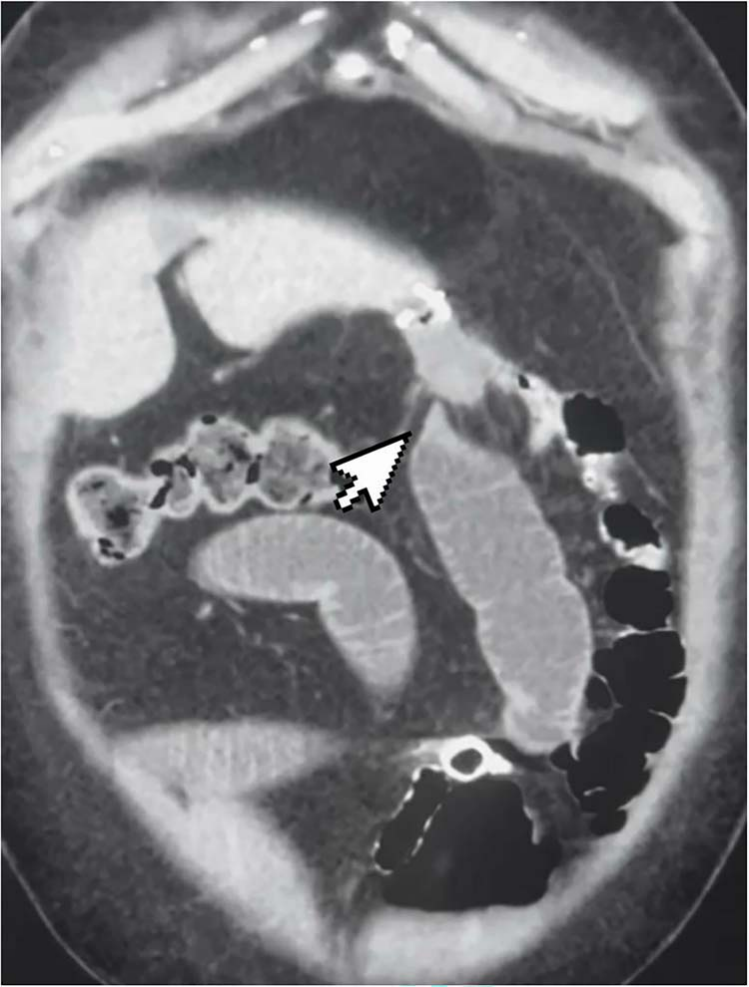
CT scan showing high-grade small bowel obstruction and twisting at the gastro-jejunal anastomosis.
